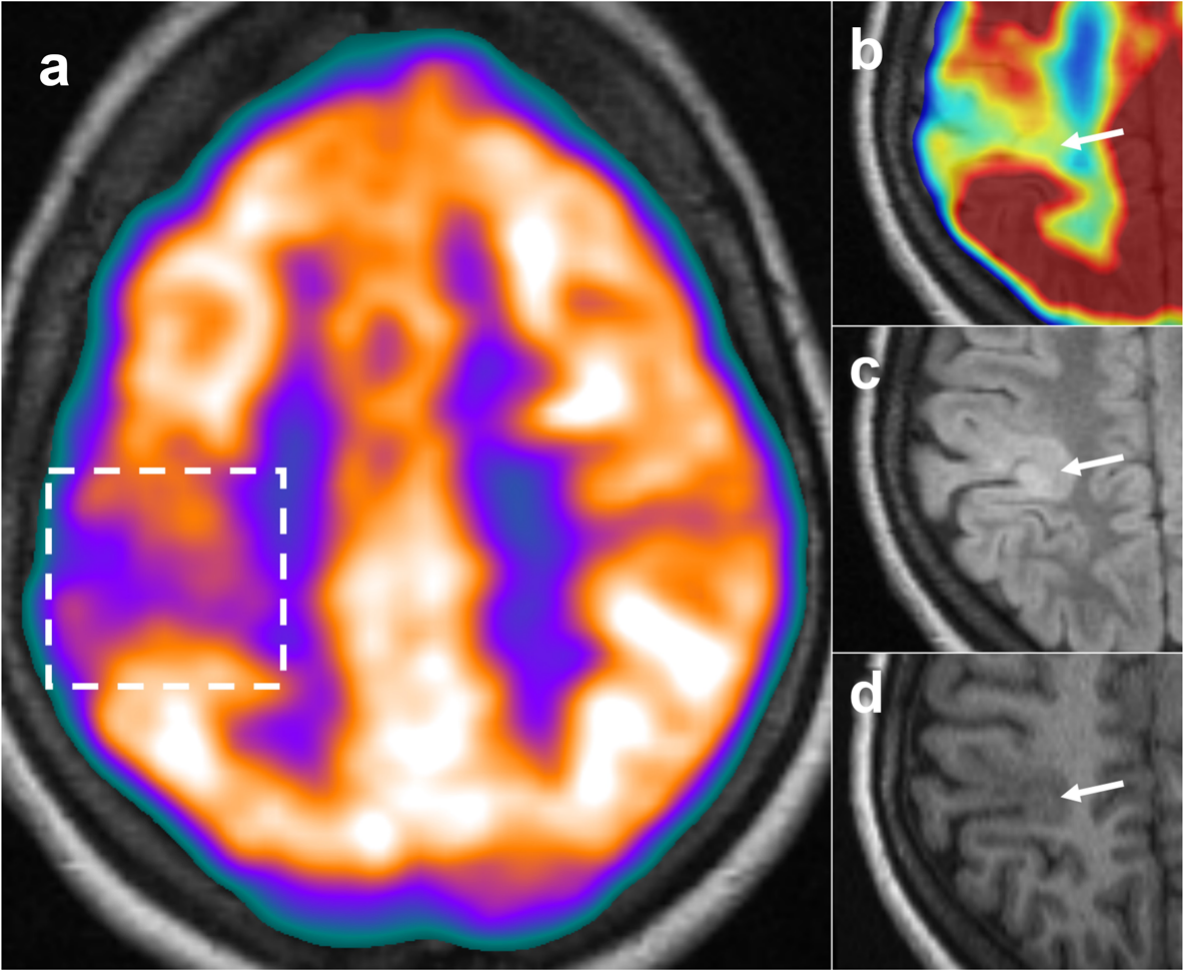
Appearance of BOSD on PET and MRI. (a) Coregistered PET and MRI data is shown for one patient who exhibited BOSD MRI hallmarks, as well as focal hypometabolism on ^18^F-FDG-PET. The area of focal hypometabolism is shown in the dashed box. The area of PET hypometabolism is shown in an inset area in (b) using a jet colour scheme. The FLAIR image (c) shows T2 hyperintensity of the BOSD, while this appears as a hypointensity on T1 (d)
